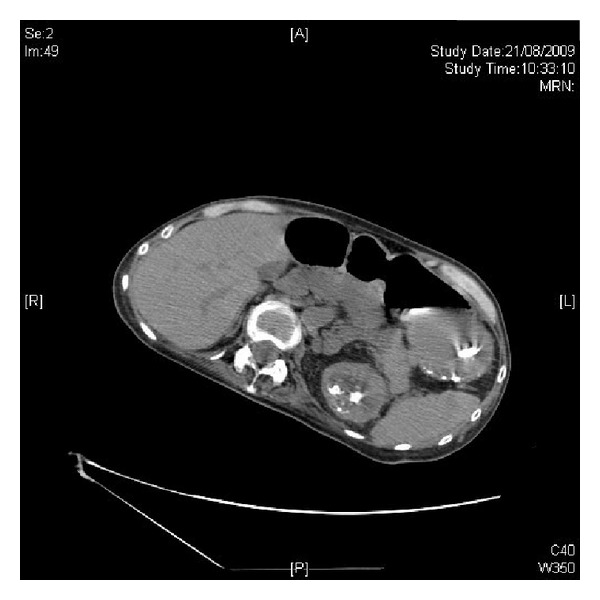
CT abdomen, performed on 21 August 2009, revealed multiple calculi in left kidney.
